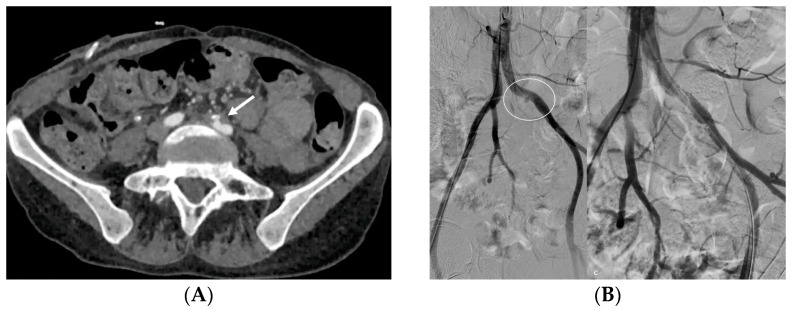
Patient with ureterocutaneostomy after a vesical resection presenting with intermittent bleeding from the cutaneous entry point. The CT study (A) demonstrates a pseudoaneurysm of a common iliac artery (arrow), confirmed also by a DSA study (B) (circle), in a point closely adjacent to the ureterocutaneostomy. DSA final control image (split image, right) after a covered stenting procedure that confirmed the complete exclusion of the pseudoaneurysm with common iliac artery patency preserved.
